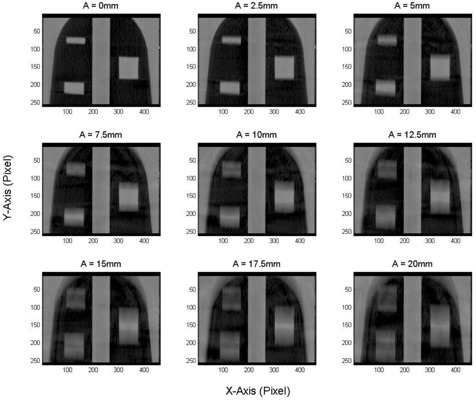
Coronal views of the CT number distributions for the three targets (small, medium, and large) with different motion amplitudes using half‐fan CBCT images. X‐ and y‐axis are represented in pixels. The targets moved cyclically in the superior–inferior direction (y‐axis) with nine different motion amplitudes: 0, 2.5, 5, 7.5, 10, 12.5, 15, 17.5, and 20 mm with a frequency of 15 cycles per min.
